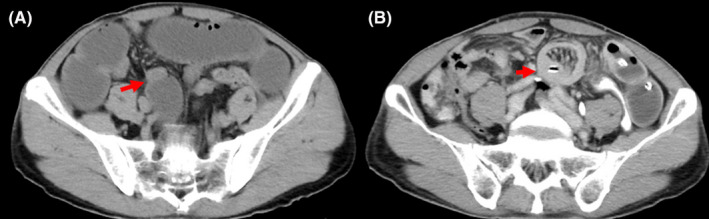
(A) Abdominal CT showing an adhesion or cord‐like structure at the portion where the caliber of the small intestine changed. (B) An enhanced abdominal CT scan showing a small bowel intussusception with a long tube in the intestinal lumen
